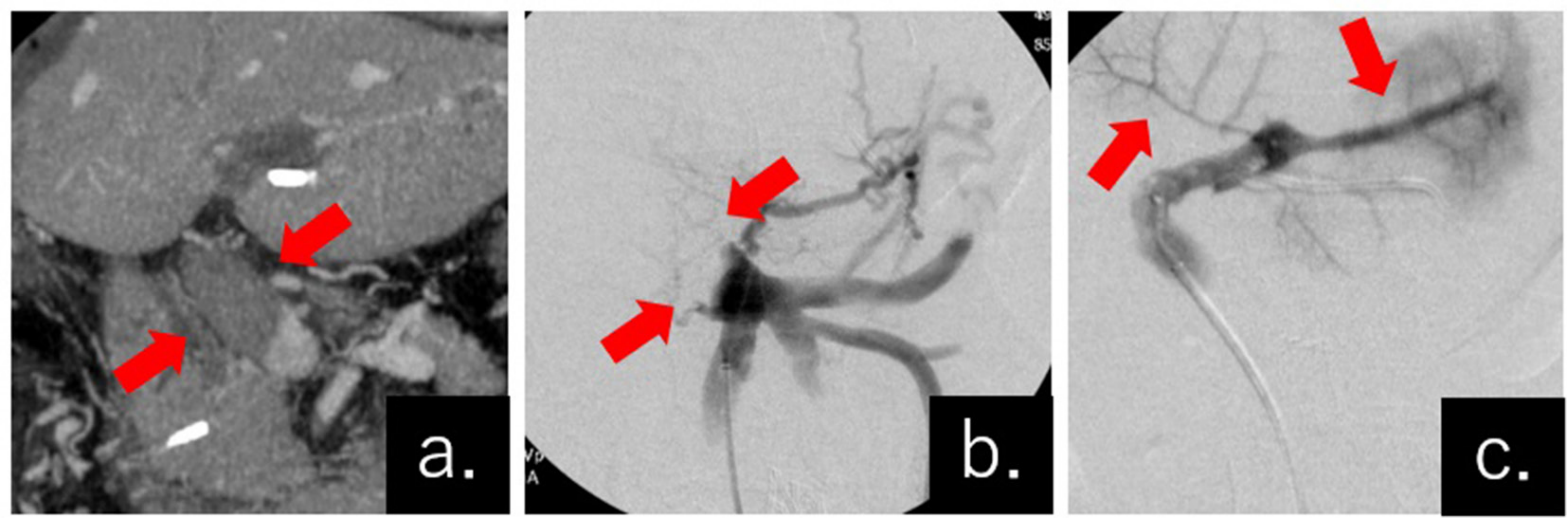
CECT of the liver after reoperation. The mesenteric catheter was placed on the distal side of the residual thrombus in the main trunk of the portal vein. CECT was displayed at 2 days (a), 9 days (b), and 16 days (c) after reoperation. Gradual thrombolysis was observed in the main trunk of the portal vein (arrows in a, b), and the residual thrombus was completely resolved 16 days after the reoperation (c). CECT, contrast-enhanced CT.
